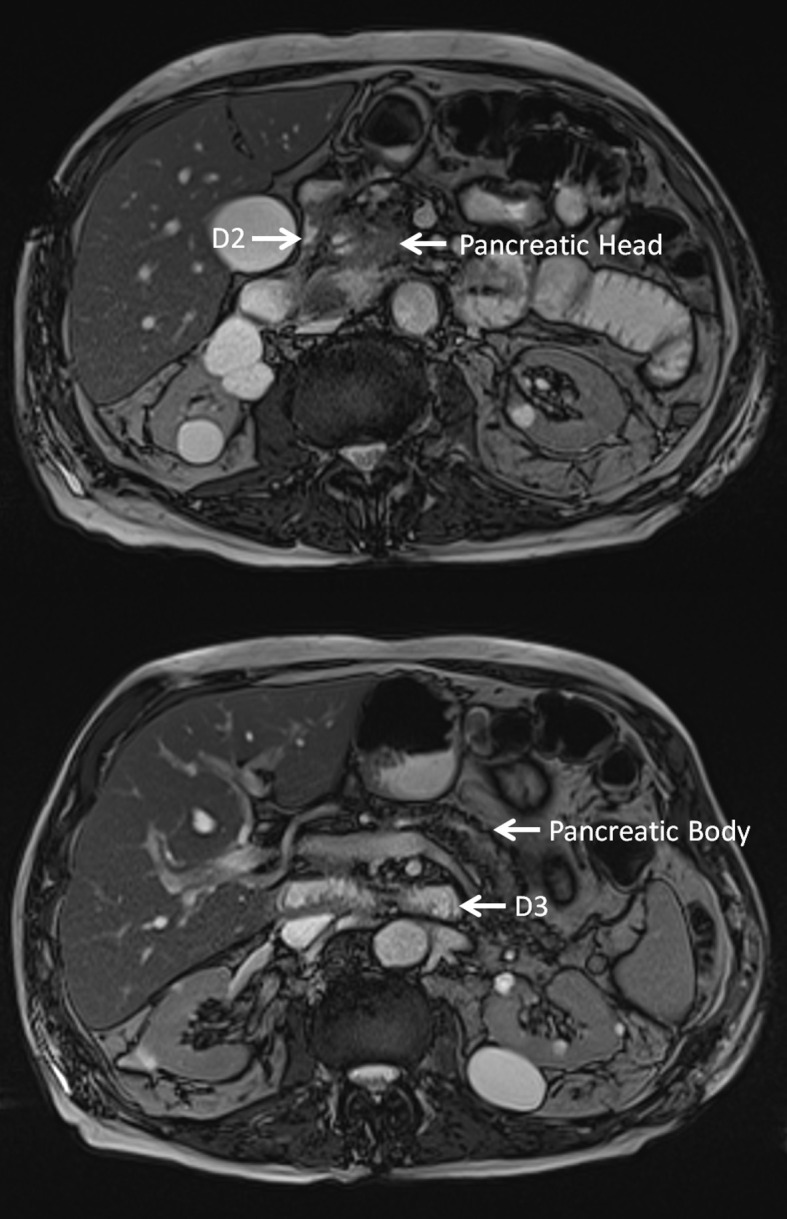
MRI TRUFI sequence axial images demonstrating the D3 segment of the duodenum at the same level of the pancreas.
